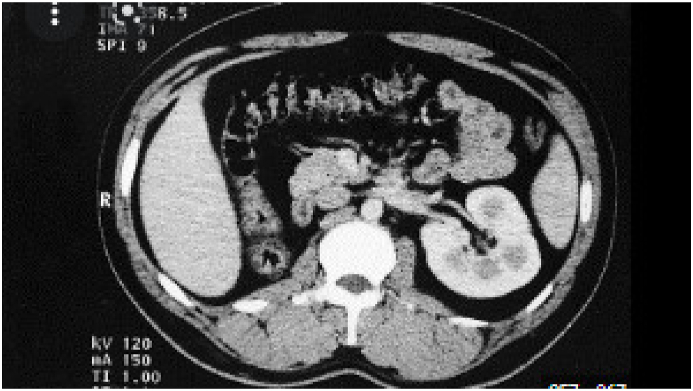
CT scan confirming right renal agenesis.
